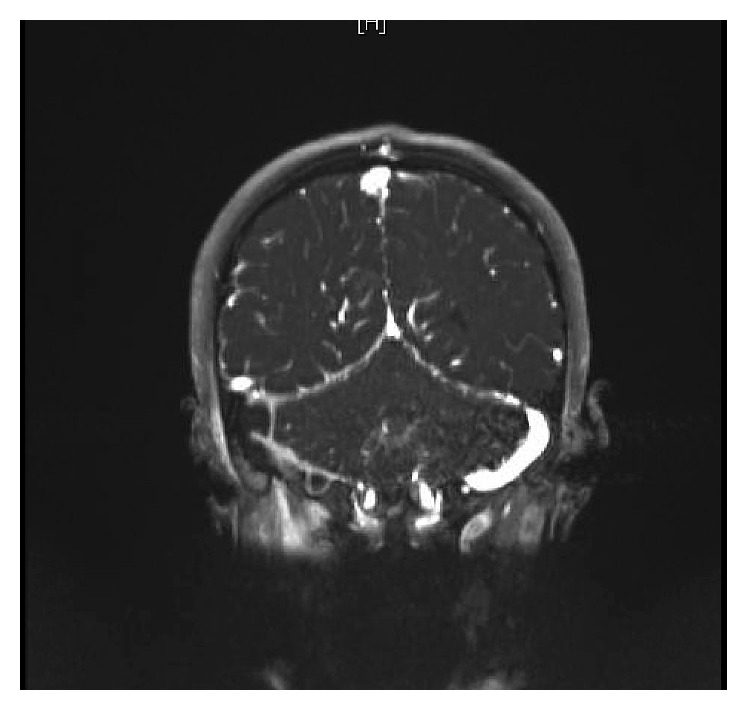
Coronal view of the MRI brain.
